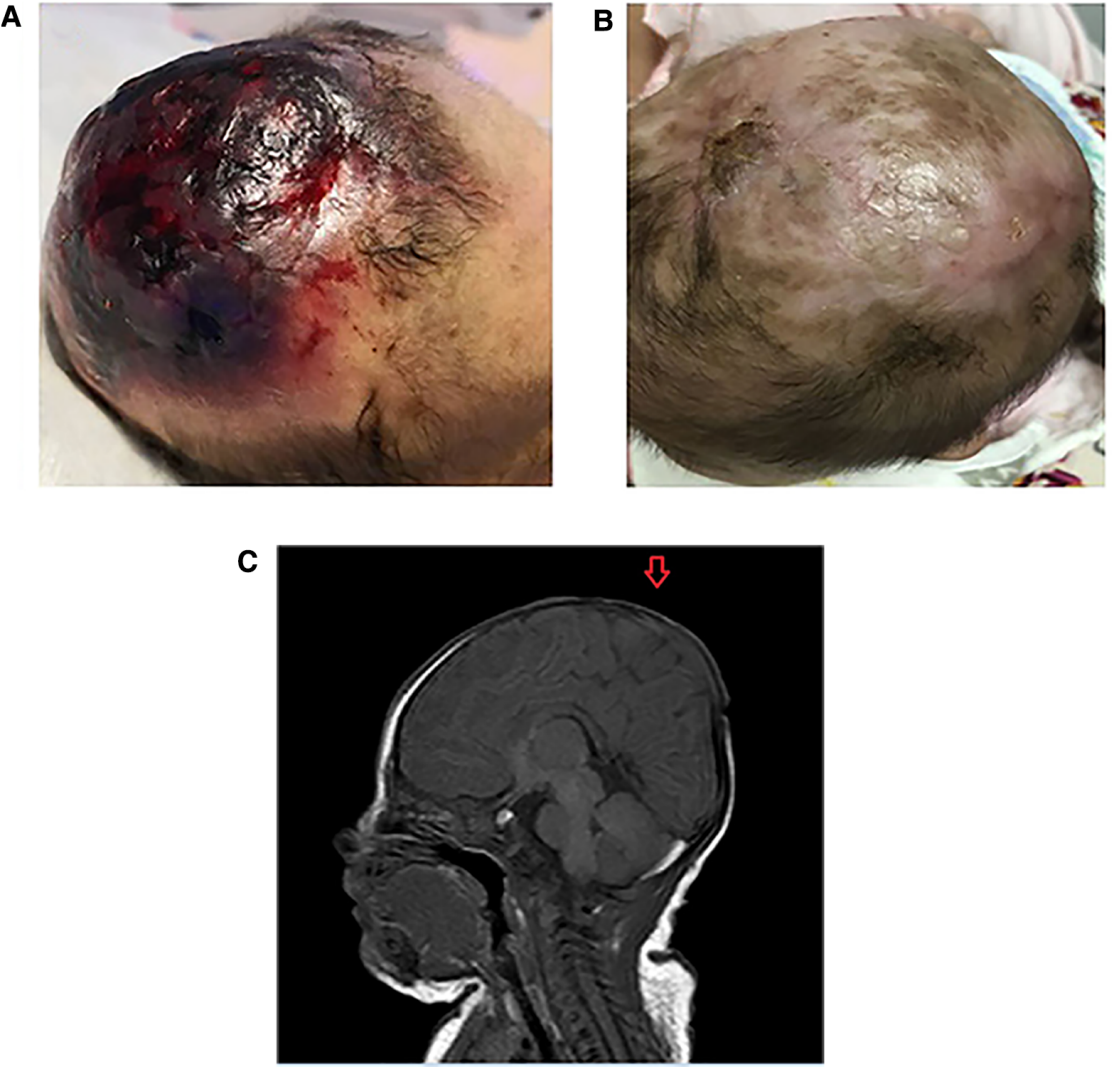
(A) The scalp defect of the patient with AOC at birth. (B) The scalp of the patient with AOC at 5 months old. (C) Cranial magnetic resonance imaging scan of the patient with aplasia cutis congenital (AOC). The scan shows a complete loss of continuity of the dermal, subcutaneous, and skull tissues (arrow).
